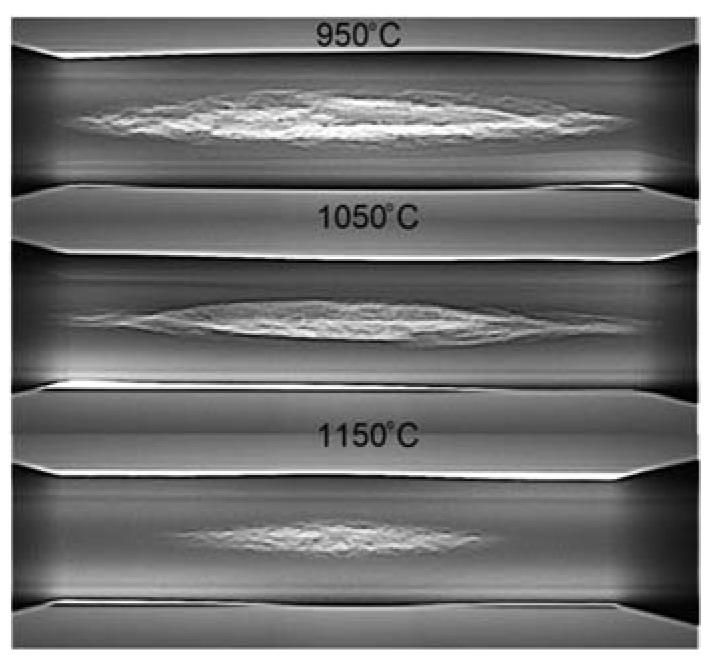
Internal cracks in the obtained forgings as shown in the X-ray radiograph.
